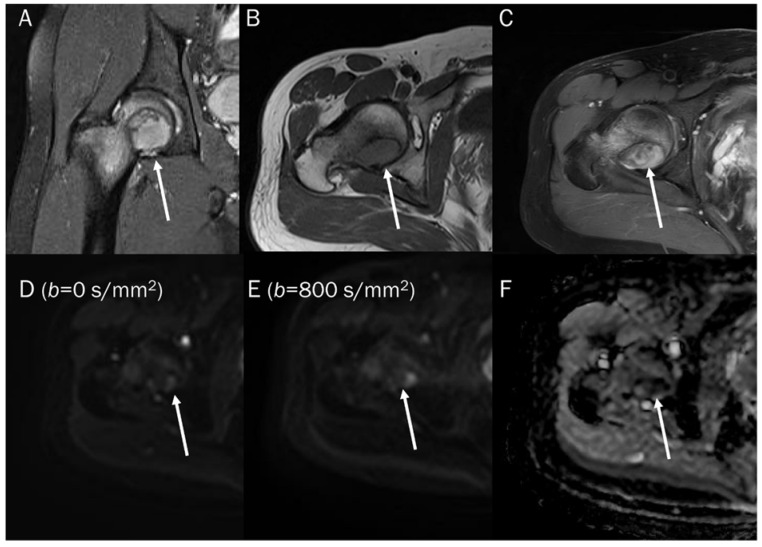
A 30-year-old male with a GCT of bone in the femoral head. Conventional MRIs (A: coronal proton-density, B: axial T1-weighted, C: axial T1-weighted enhanced) show a well-circumscribed mass (arrow) with proton-density high and T1 intermediate signal intensity, as well as heterogeneous enhancement in the femoral head, accompanied by extensive peritumoral edema, raising the possibility of a malignant bone tumor. (D,E) DWI with *b*-values of 50 and 800 s/mm^2^ exhibit persistent low signal (arrow) with different *b*-values, while (F) the ADC map shows a signal drop (arrow), indicating a T2 “black-out” effect, which could be attributed to susceptibility artifacts of hemosiderin.
